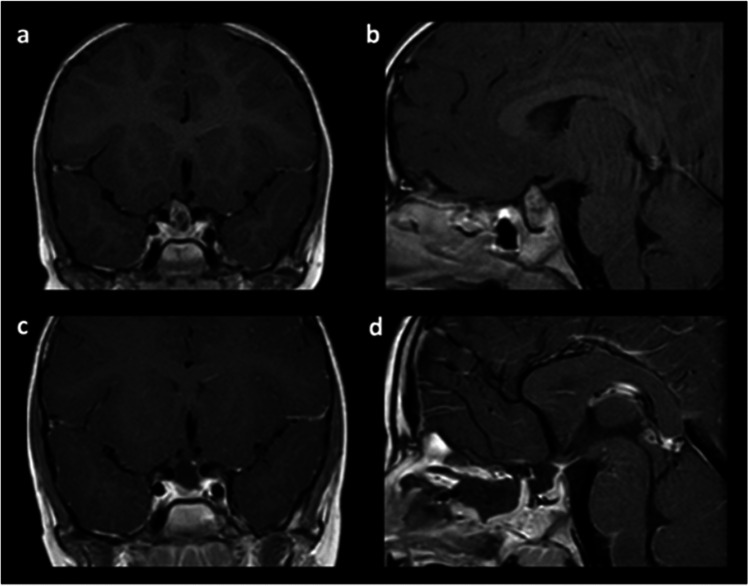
Sellar and suprasellar adamantinomatous craniopharyngioma in a 4-year-old female: a, b coronal and sagittal T1-weighted contrast-enhanced preoperative MRI; c, d coronal and sagittal postoperative MRI, showing gross total resection
